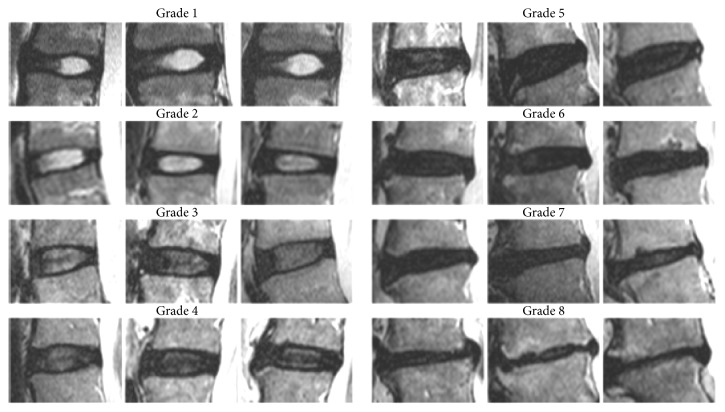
MRI images of the Modified Grading System for Lumbar Disc Degeneration. This material is used after author's permission [17].
